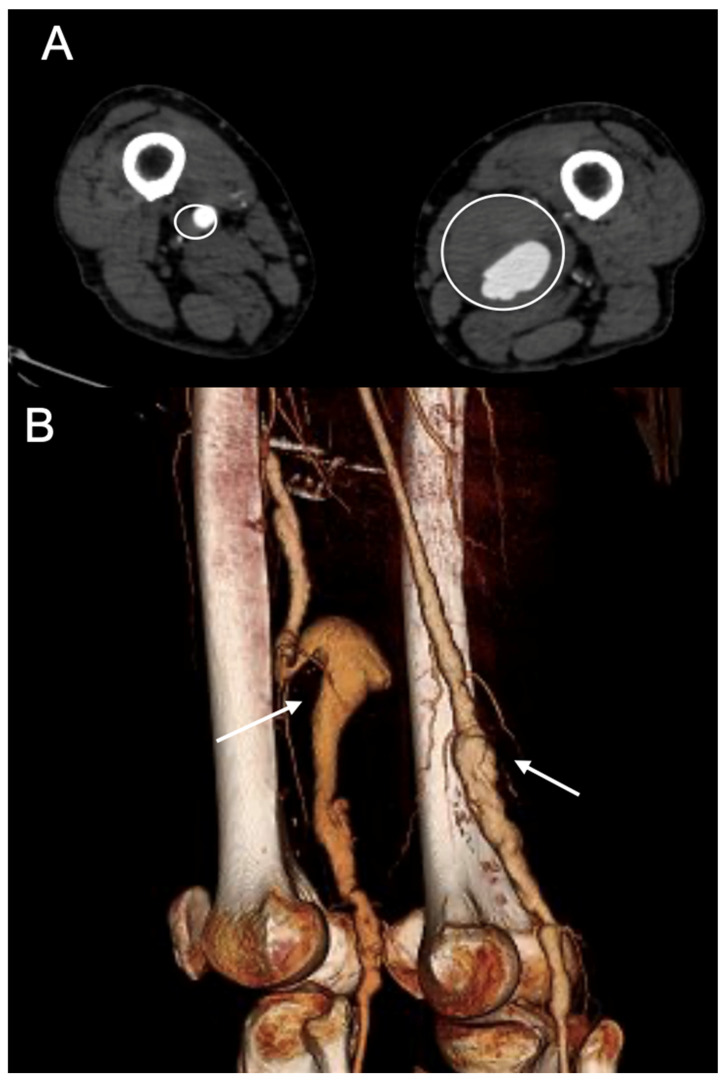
Preoperative computed tomography angiography of a patient presenting with a bilateral PAAA treated at our institution. (A) A case of bilateral popliteal artery aneurysm (PAA) treated at our institution. A transversal slice of the preoperative CTA (circles) and a 3D reconstruction (B) (arrows).
